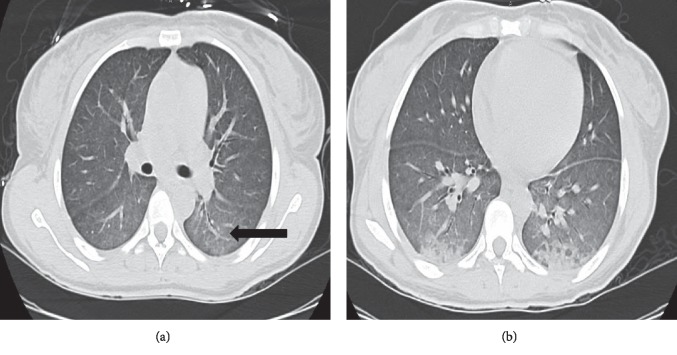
(a, b) CT scan of the chest showed lower lobe predominant confluent ground-glass opacities (arrow).
